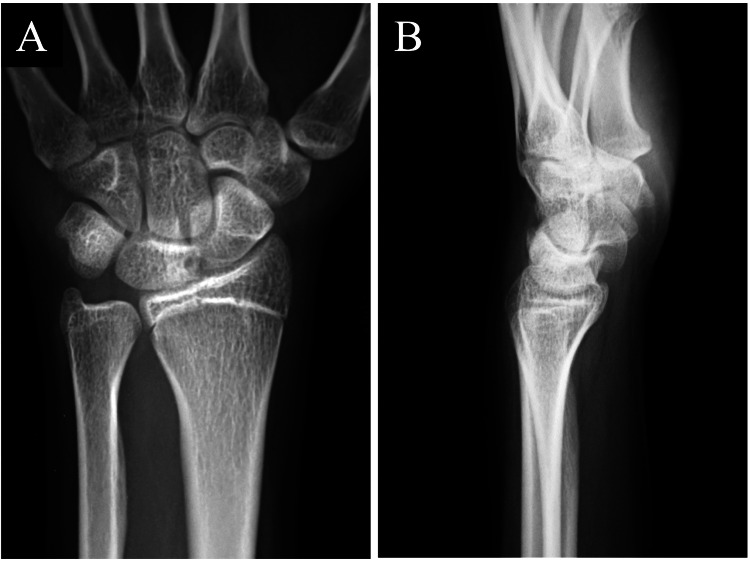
Preoperative radiographs of the left wrist. (A) Posteroanterior view. (B) Lateral view.
